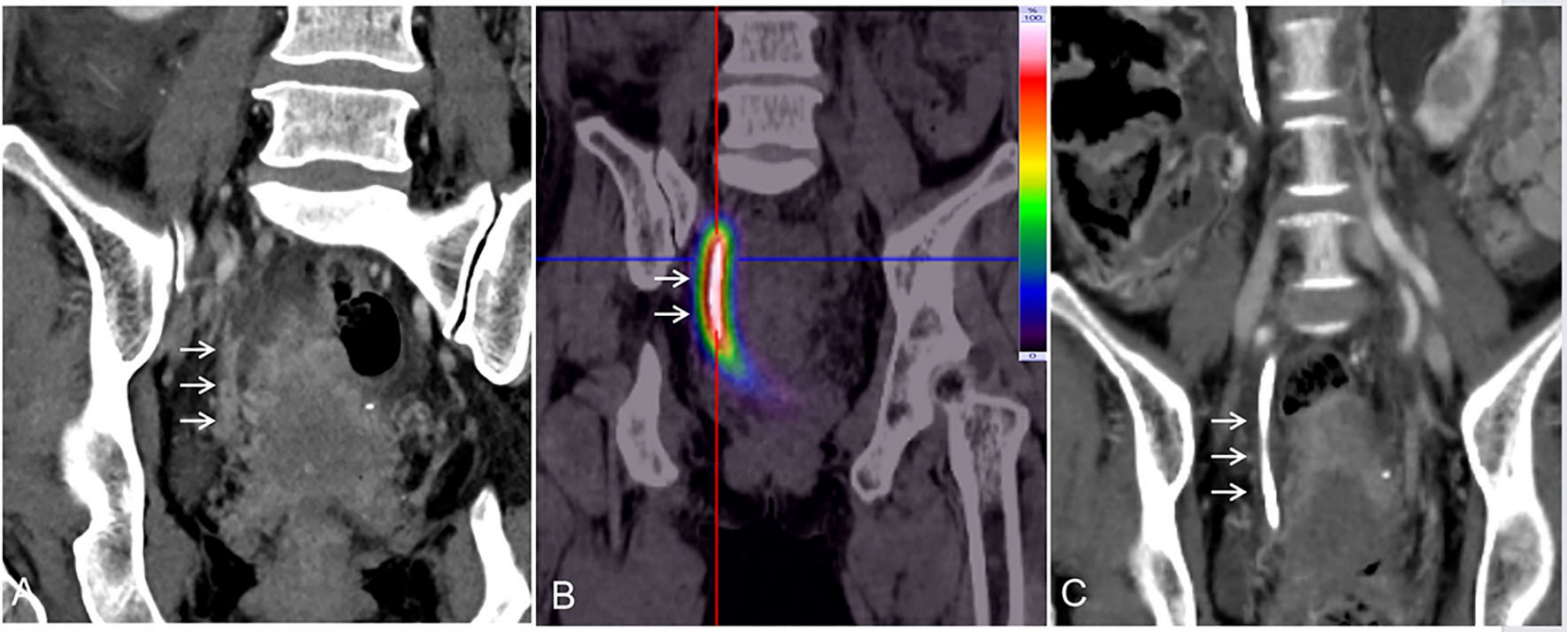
(A) Preoperative enhanced CT image showing thickening of the right lower ureteral wall (arrow) and ureteral carcinoma confirmed by forceps biopsy. (B) After implantation of this novel BPS, SPECT revealed a local γ-ray distribution (arrow), and the surrounding normal organ radiation dose was extremely low. (C) After two months of brachytherapy, the local tumor disappeared (arrow), and the expansion of the collection system disappeared.
